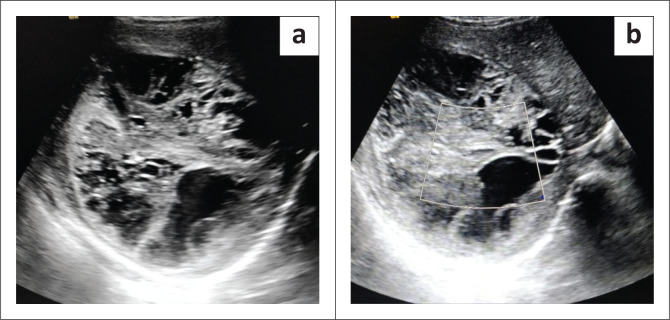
(a) A well-defined heterogeneous lesion in the right lobe of liver with solid echogenic areas and anechoic cystic areas; (b) No obvious internal vascularity on Doppler imaging.
